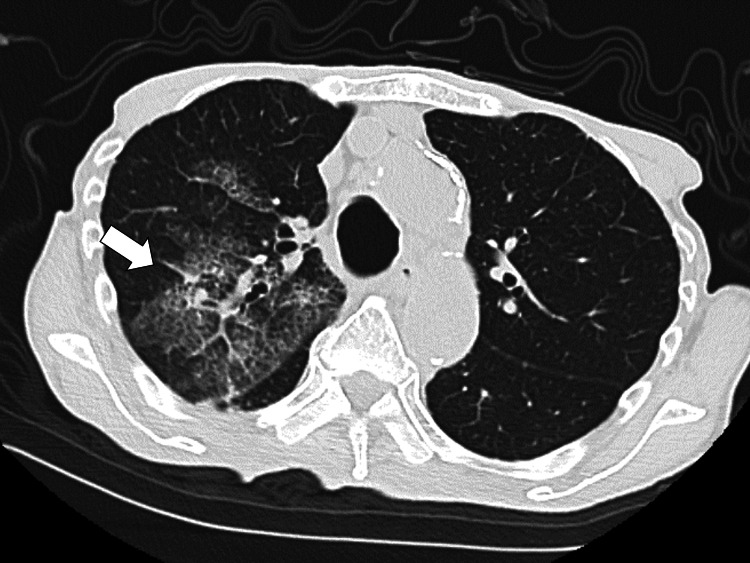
Computed tomography of the chest showing multiple lesions of ground-glass opacities on bilateral lungs showing alveolar hemorrhage (white arrow)
